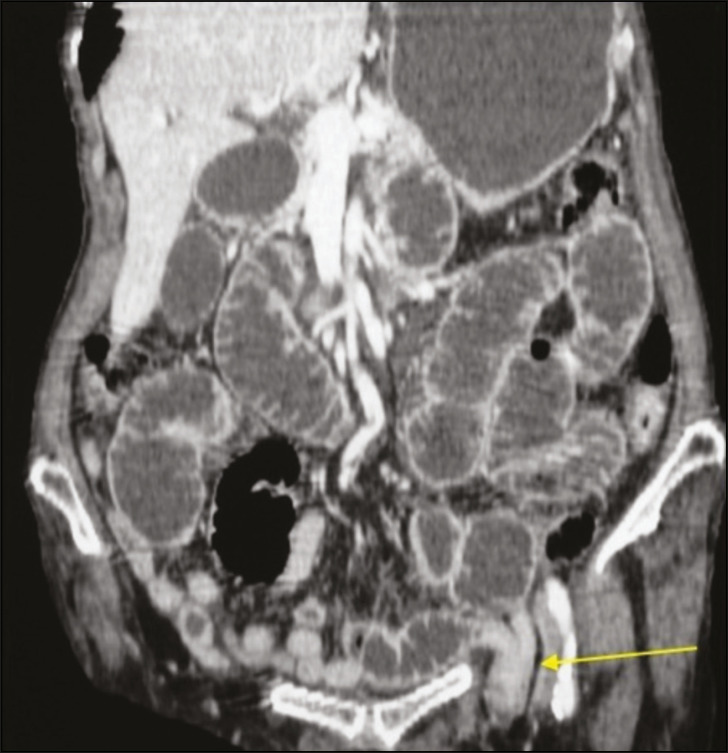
Coronal CT in the portal phase showing an incarcerated left inguinal hernia containing a jejunal loop, complicated by intestinal obstruction. Note the distention of the proximal bowel loops.
